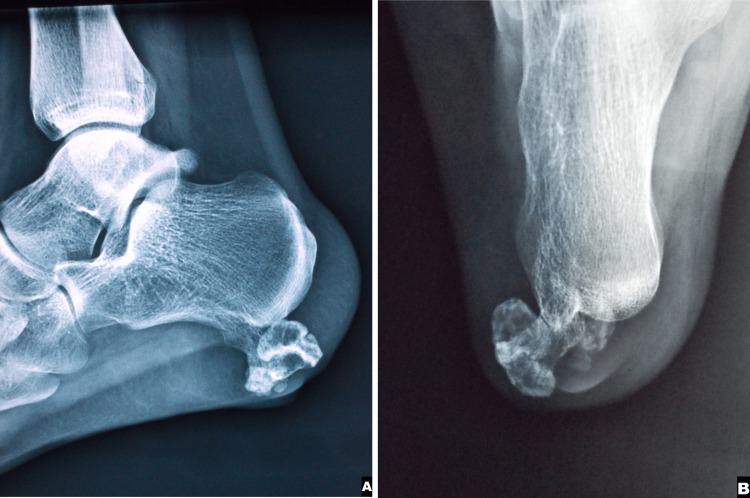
Lateral and axial ankle views Figure 1A: A lateral X-ray demonstrating the posteroinferior location of the tumor and its large exophytic osseous growth; Figure 1B: An axial radiograph reveals its origin in the lateral process of the calcaneal tuberosity. Appearance is compatible with an osteochondroma.
